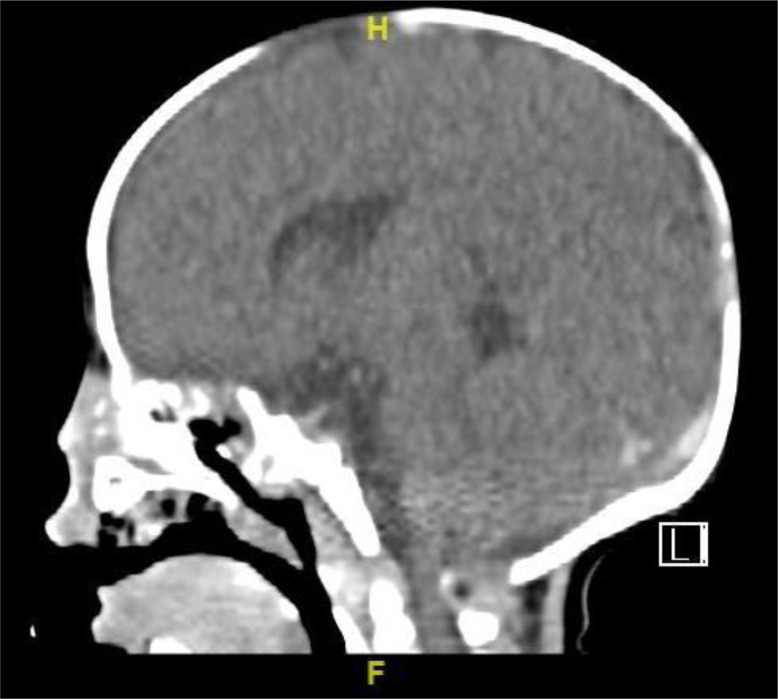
CT imaging (Day 45) demonstrating advanced invasive Aspergillus flavus sinusitis with complete craniofacial destruction involving nasal bones, hard palate, and bilateral maxillary structures.
